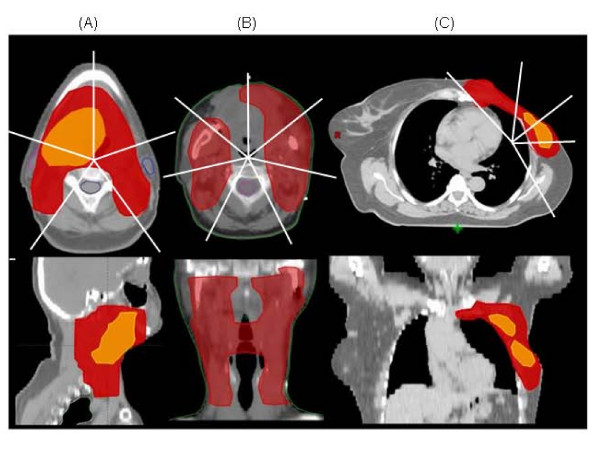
The three planning cases selected for the study. (A) base of tongue, (B) mandible, (C) left thoracic wal. An axial CT slice approximately at the centre of the target volume is shown together with a reconstructed coronal or sagittal view; target volumes are shown as overlays.
